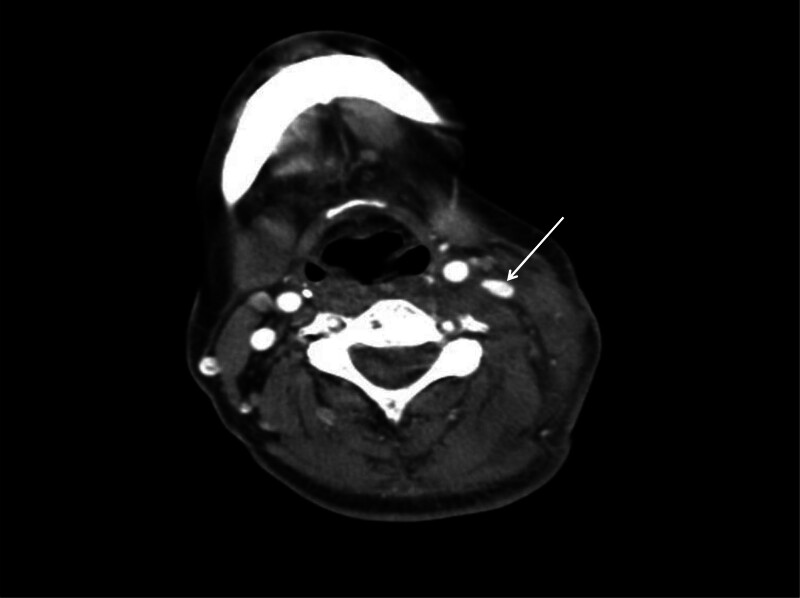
Postoperative enhanced CT at the neck, with the arrow indicating a narrowing of the repaired internal jugular vein diameter and unobstructed blood flow. CT = computed tomography.
